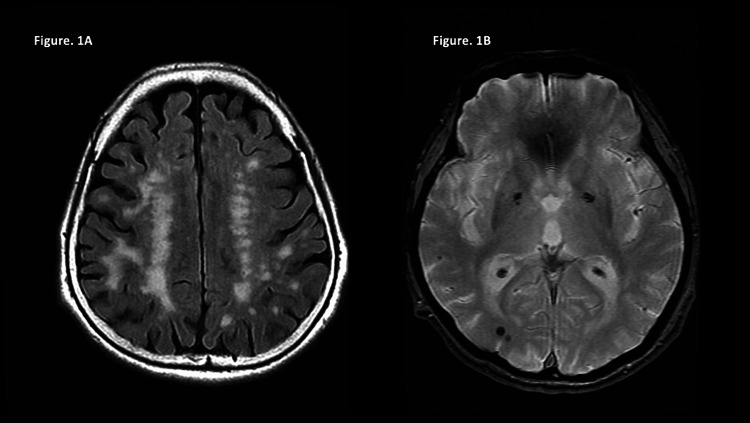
Brain MRI. (A) High signal intensity can be seen in the bilateral cerebral white matter, indicating chronic ischemic changes on T2WI/FLAIR. (B) Low signal intensity can be seen in bilateral temporal lobes, occipital lobes, right subcortical frontal lobes, and right illuminating hemisphere, indicating obsolete microbleeds and CAA on T2WI. CAA: cerebral amyloid angiopathy; T2WI: T2-weighted image; FLAIR: fluid-attenuated inversion recovery
